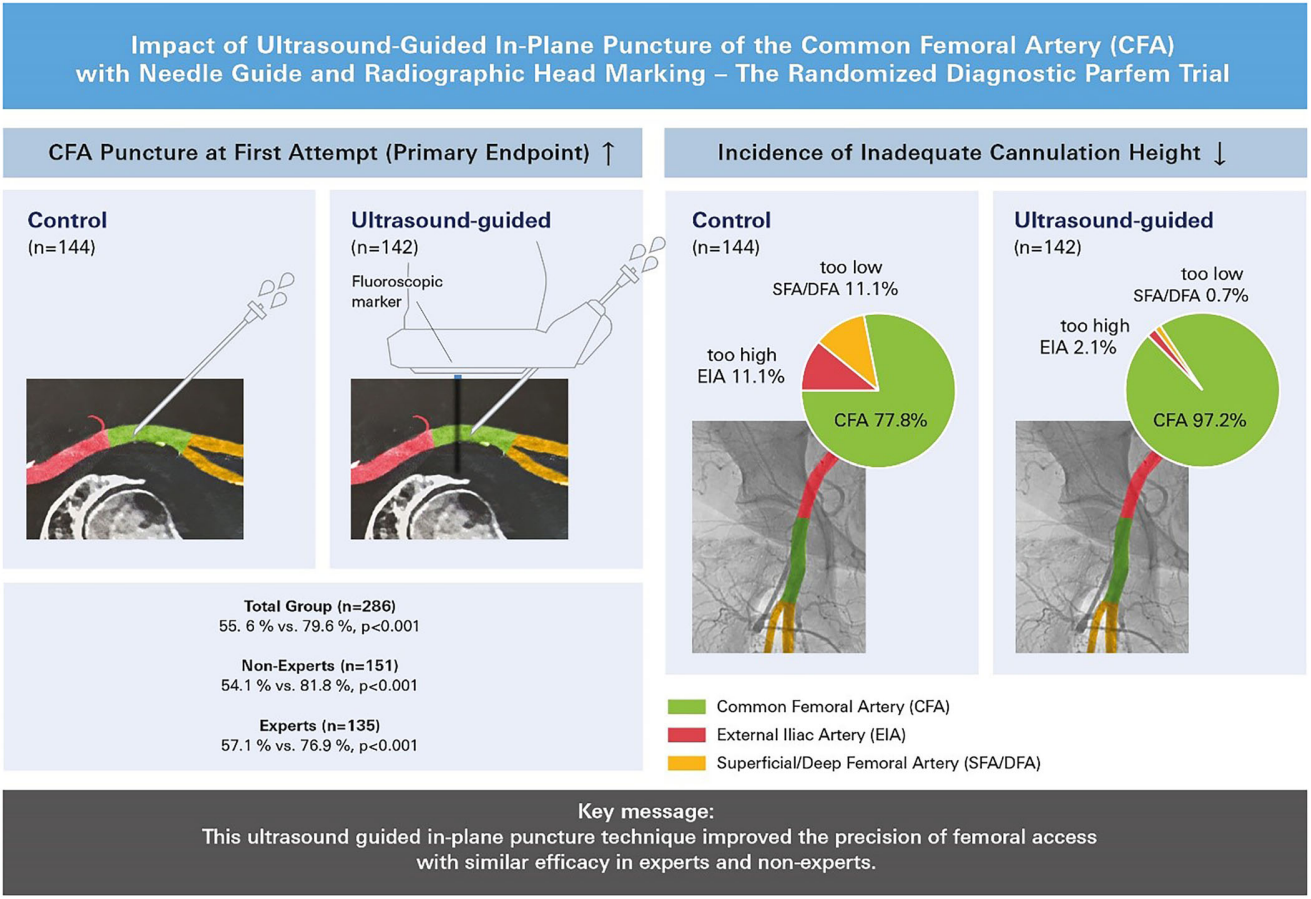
Ultrasound guided in‐plane puncture of femoral artery (PARFEM‐Trial). [Color figure can be viewed at wileyonlinelibrary.com]
